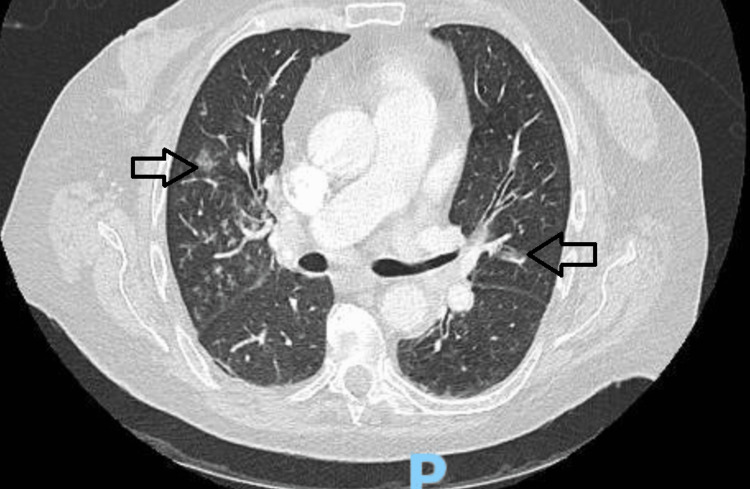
Computed tomography of the chest Bilateral patchy nodular areas of ground-glass opacifications (arrowheads) in the lung parenchyma.
